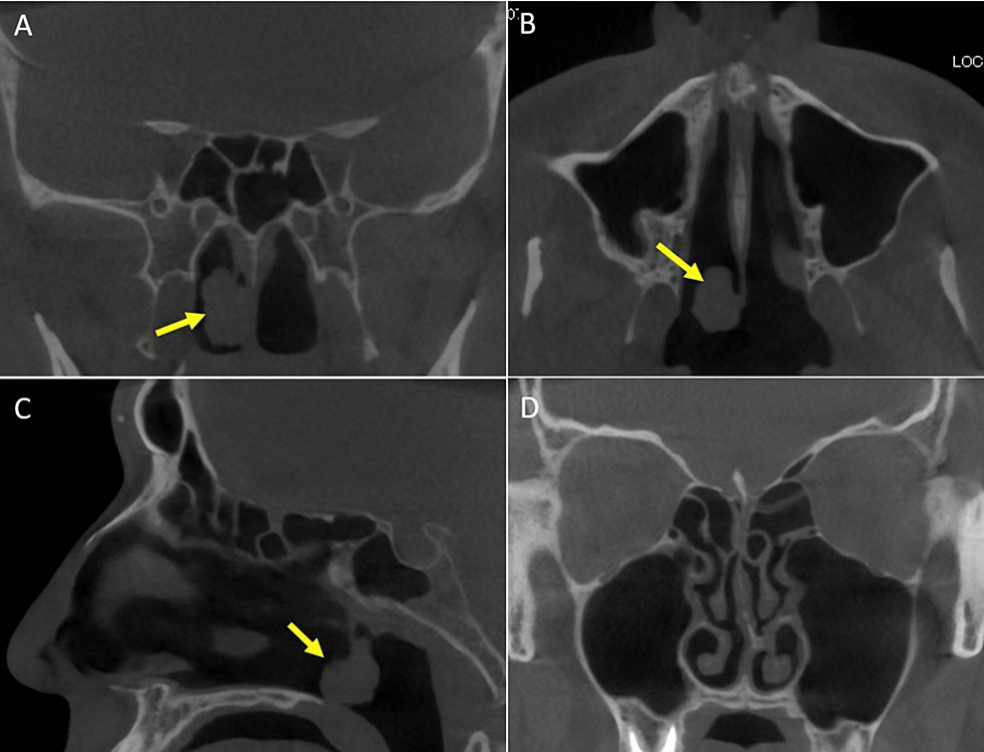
Computed tomography findings. A coronal image B. axial image C. sagittal image (A) coronal image (B) axial image (C) sagittal image. A soft tissue mass is derived from the right nasal septum (yellow arrows in images A, B, and C). Bone destruction and sinus invasion are not confirmed. D. Nasal septum deviates to the left side (intact side).
